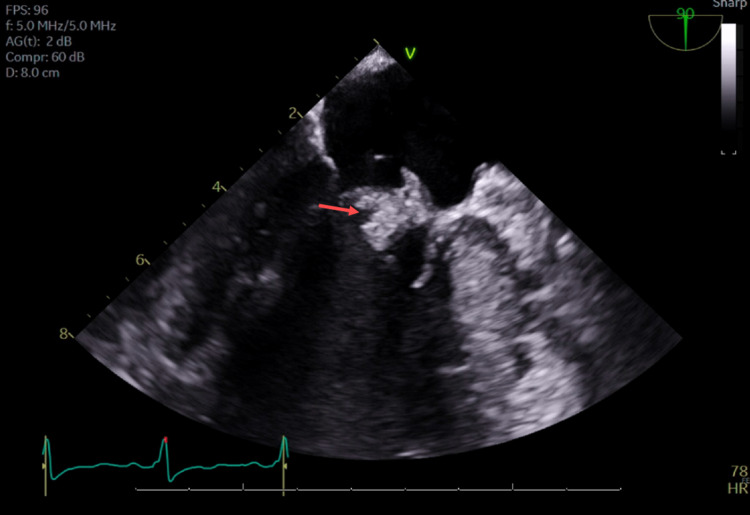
Transesophageal echocardiography visualizing the mitral valve vegetation (red arrow) measuring 1.5 cm x 0.9 cm attached to the anterior mitral leaflet on the ventricular side and prolapsing to the atrial side and left ventricular outflow tract. A moderate eccentric posterior jet of mitral regurgitation was noted. Other pertinent transesophageal echocardiographic findings included normal left ventricular systolic function with an estimated left ventricular ejection fraction of 65%-70%, significant left ventricular hypertrophy with systolic anterior motion of the anterior mitral leaflet, a defibrillator wire in the right atrium and right ventricle with no suspicious vegetation or thickening, normal tricuspid aortic valve, no evidence of intracardiac shunting with an agitated saline bubble study, and a clear left atrial appendage with no evidence of a thrombus.
